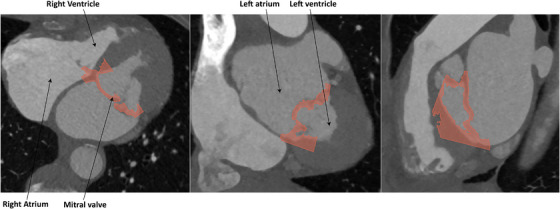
Fusion overlay after the semiautomatic algorithm. A TEE segmentation of the mitral valve is transparently visualized as an overlay over CT slices.
